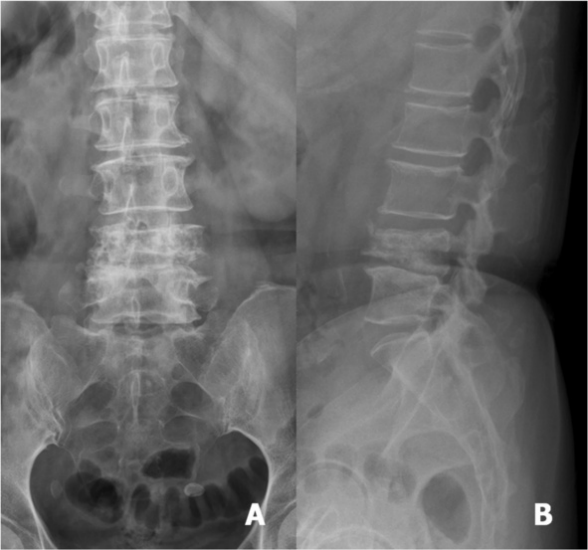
A 57-year-old woman had breast cancer with L4 metastasis. Anteroposterior (a) and lateral radiograph (b) revealed a collapse of the vertebral body of L4
